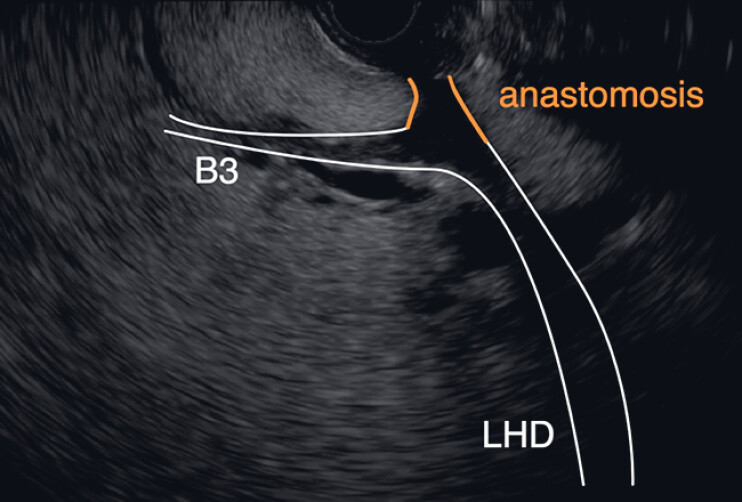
Endoscopic ultrasound findings. A hypoechoic area consistent with the anastomosis was identified in continuity with the bile duct. LHD, left hepatic duct.
